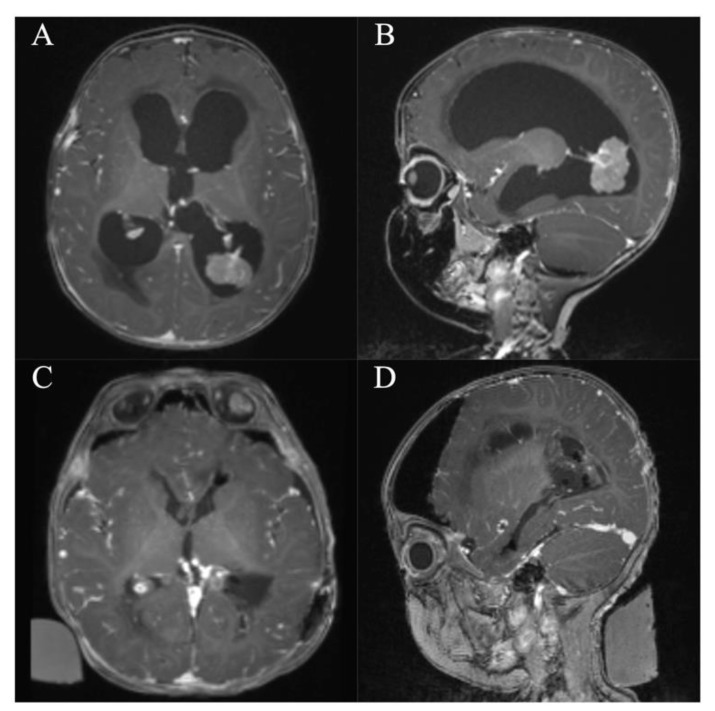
Case 6. Axial and sagittal MR images, T1-weighted sequences with contrast medium preoperatively (A,B) and immediately postoperatively (C,D) after resection of the intraventricular choroid plexus carcinoma via a left occipital endoscopic-assisted approach.
